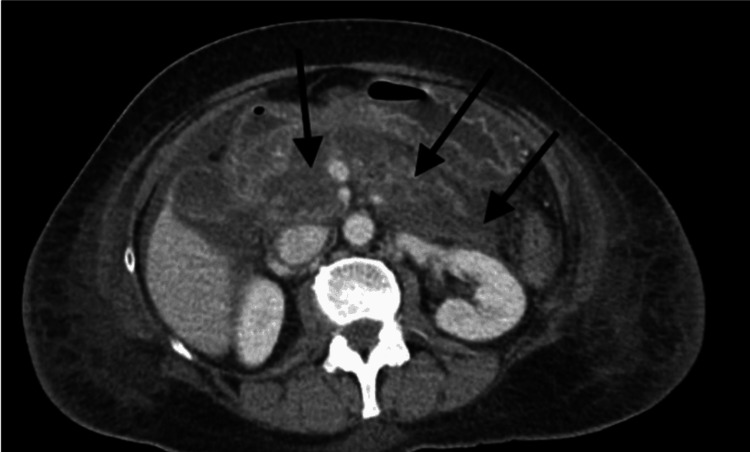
CT imaging confirming acute necrotizing pancreatitis. Arrows pointing to areas of acute edema and necrosis, confirming the presence of acute necrotizing pancreatitis hours after the use of intravenous lipid infusion.
